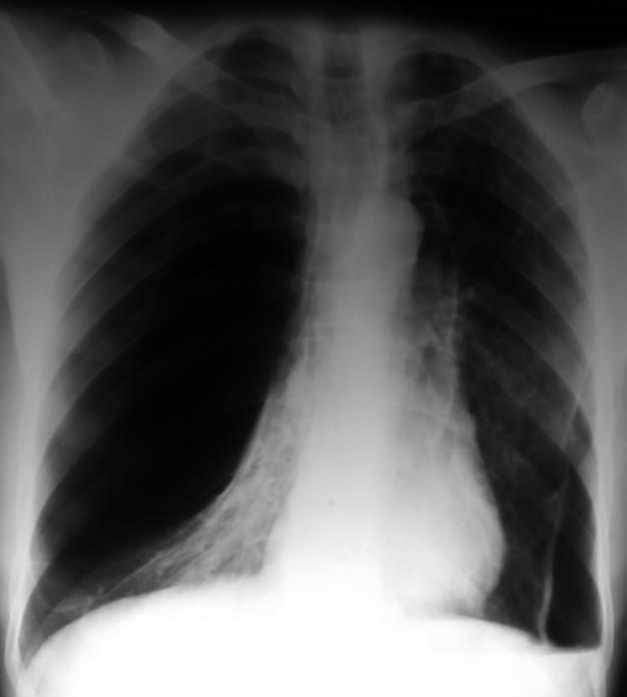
Radiographie du thorax montrant une bulle d’emphysème géante droite
